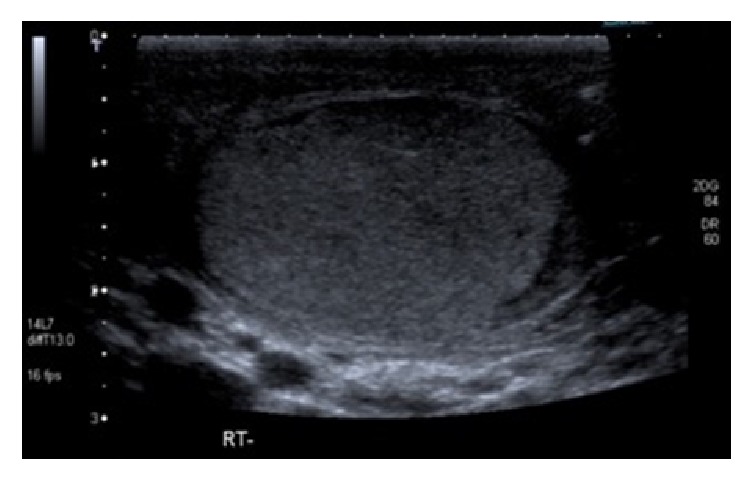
Follow-up ultrasound scan of right testis with a small amount of residual haematoma in scrotal sac.
